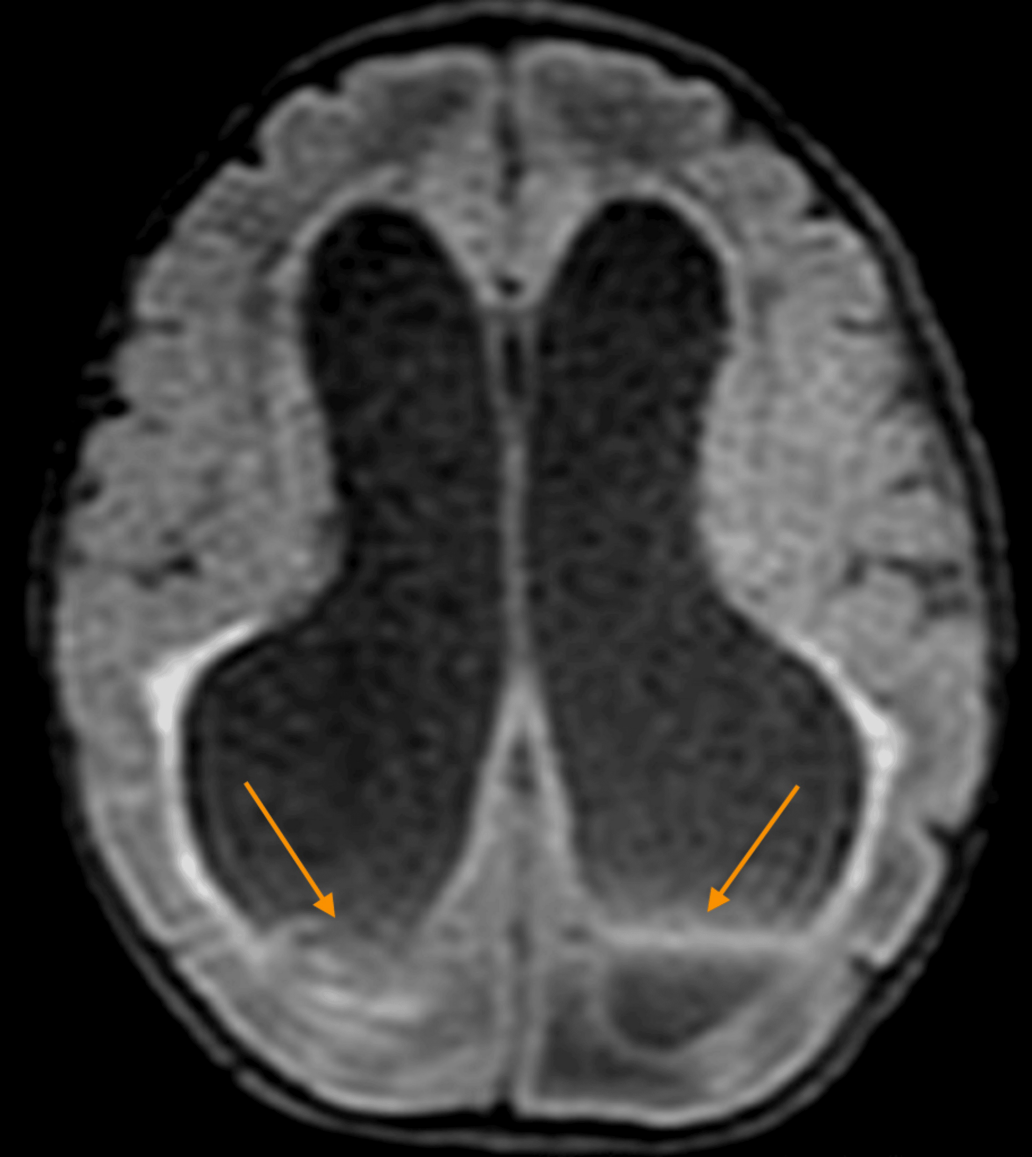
Axial T2 FLAIR MRI demonstrating a grade 3 intraventricular hemorrhage with associated ventriculomegaly (Case 1). Arrows show blood within the occipital horns of both ventricles, consistent with extensive bleeding and impaired cerebrospinal fluid drainage. FLAIR: fluid-attenuated inversion recovery
